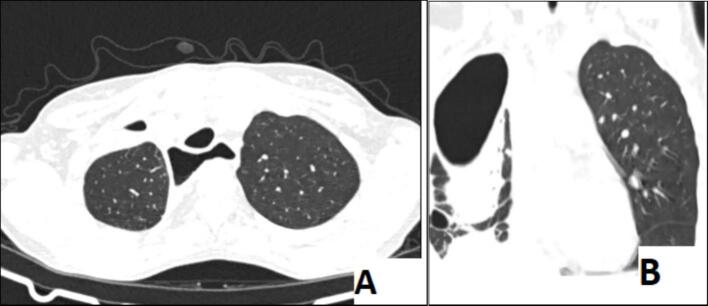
A (Axial), B (Sagittal), and C (Coronal) follow-up thoraco abdominal contrast-enhanced CT scan demonstrated decreased right pleural effusion, reduced size of the right lower lobe cavitary lesion with surrounding consolidation, and resolution of multifocal right lung opacities. The esophagus remains dilated with reduced intraluminal stasis.
